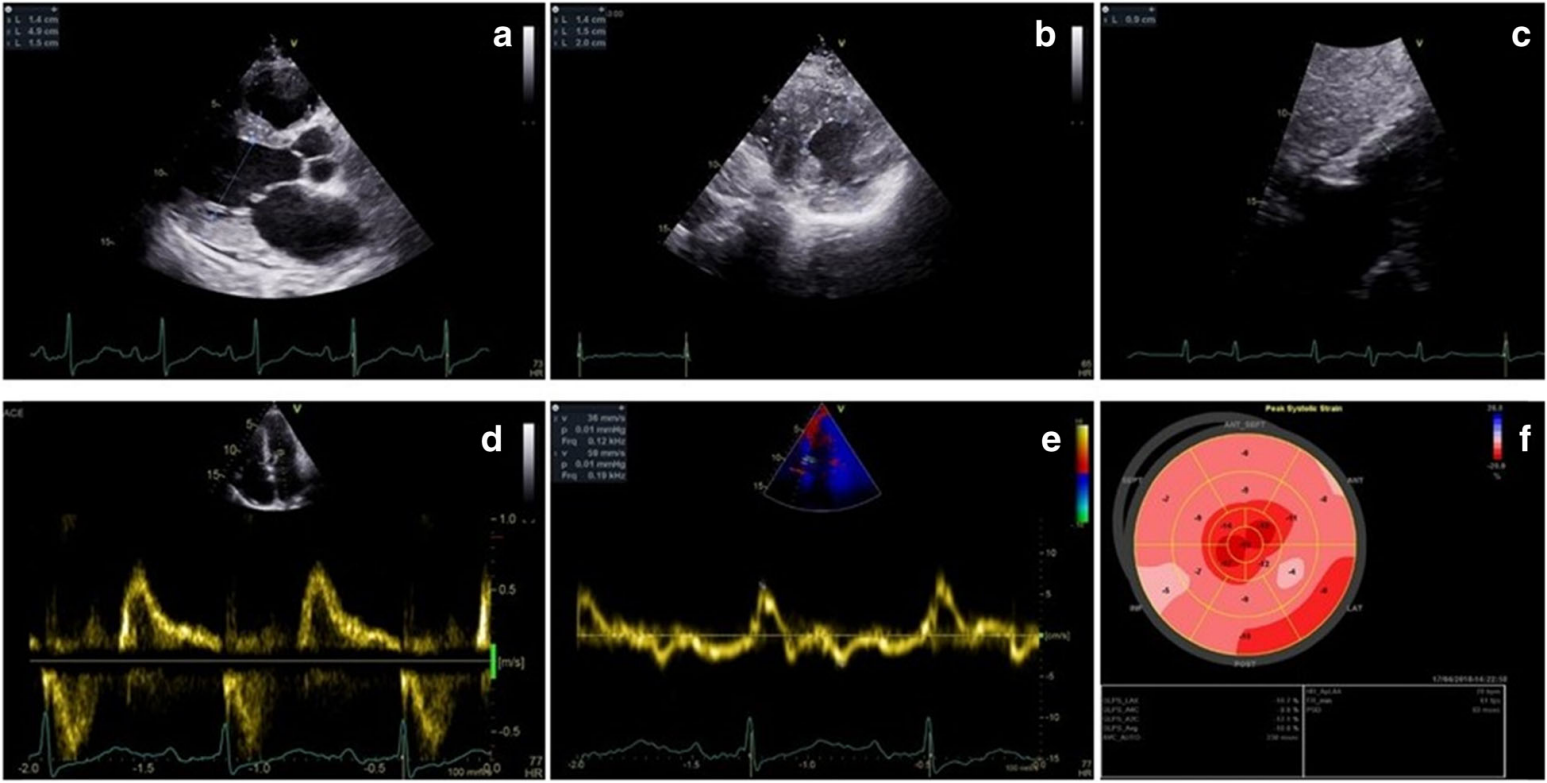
Transthoracic 2D echocardiography. a. Parasternal long-axis section revealing concentric LV hypertrophy. b. Parasternal midventricular short axis with maximal wall thickness of 20 mm at inferoseptal level. c. Subcostal view with RV free wall hypertrophy (9 mm). d. Transmitral flow Doppler interrogation finds grade 3 diastolic dysfunction. e. Myocardial Doppler interrogation of the septal mitral annulus shows low longitudinal systolic and diastolic velocities. f. Bull’s eye LV myocardial deformation map with low global longitudinal strain and apical sparring
